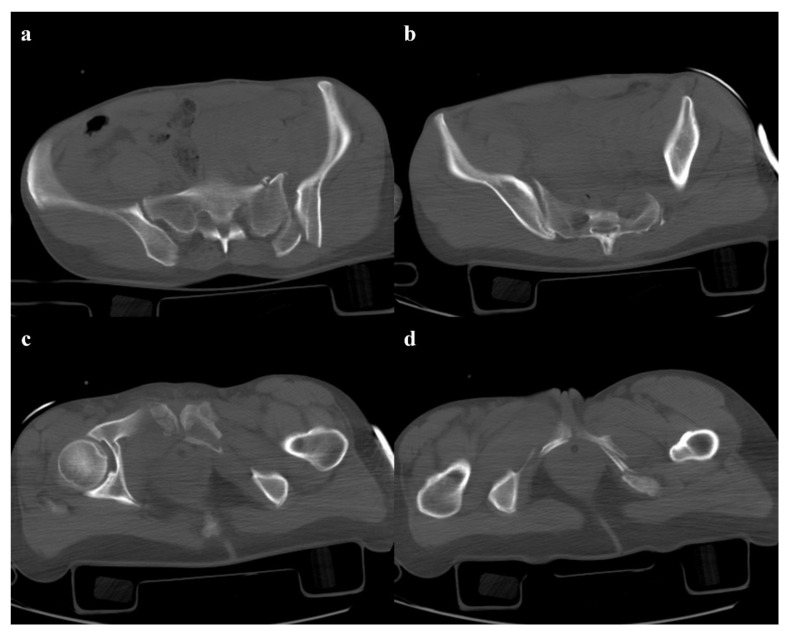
Lateral compression fracture, type 3. Axial CT images show the fracture of the iliac and sacral wings on the left side (a), anterior widening of the right sacroiliac joint (b), and fracture of bilateral pubic branches (c,d).
